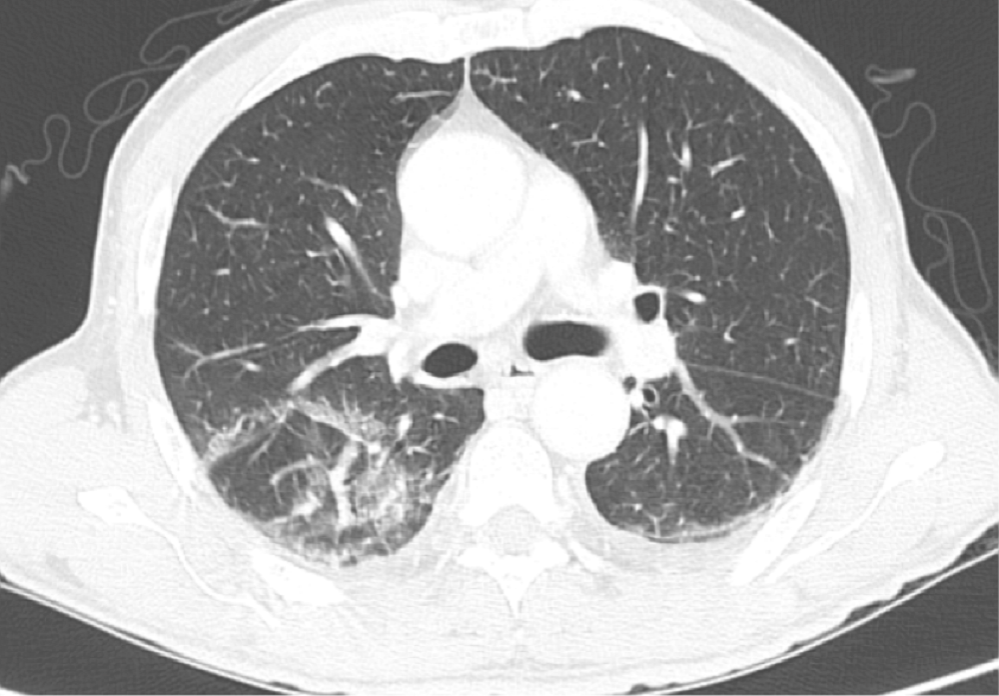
Computed tomography of the chest on postoperative day 4.
